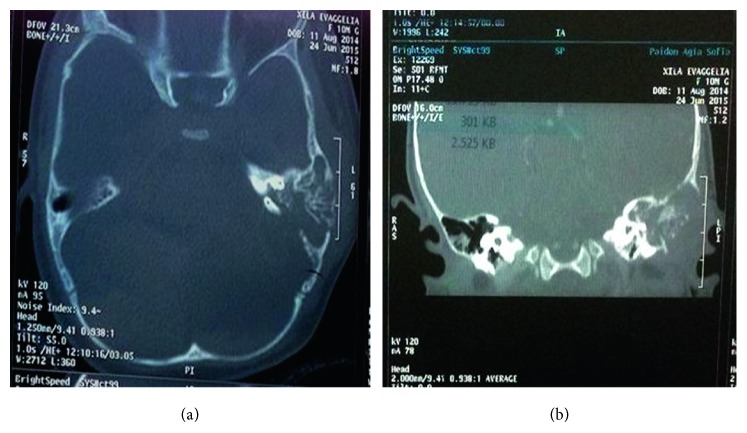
CT of the temporal bone. An invasive lesion of the mastoid cavity on the left with widespread corrosion of the trabeculae of the bone expanding towards the cranial bones and the underlying meninx.
